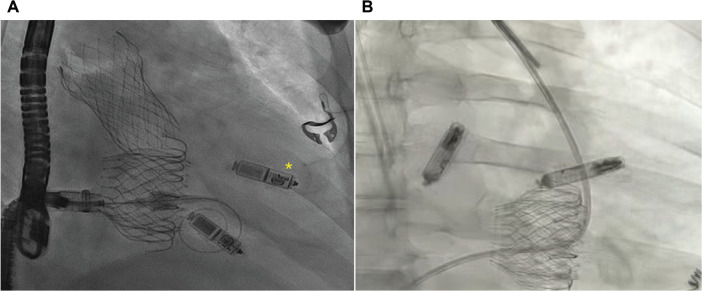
Frontal chest radiographs of patients with pre-existing leadless pacemakers undergoing transcatheter tricuspid valve replacement (TTVR). A: Deployment of a TTVR in a patient with two Micra™ devices. The mid-septal device (asterisk) is the more recent implant. B: A TTVR implanted in a patient with an AVEIR DR. The ventricular AVEIR is implanted in the mid-high septum, and there is clear interaction between the valve and the leadless device.
